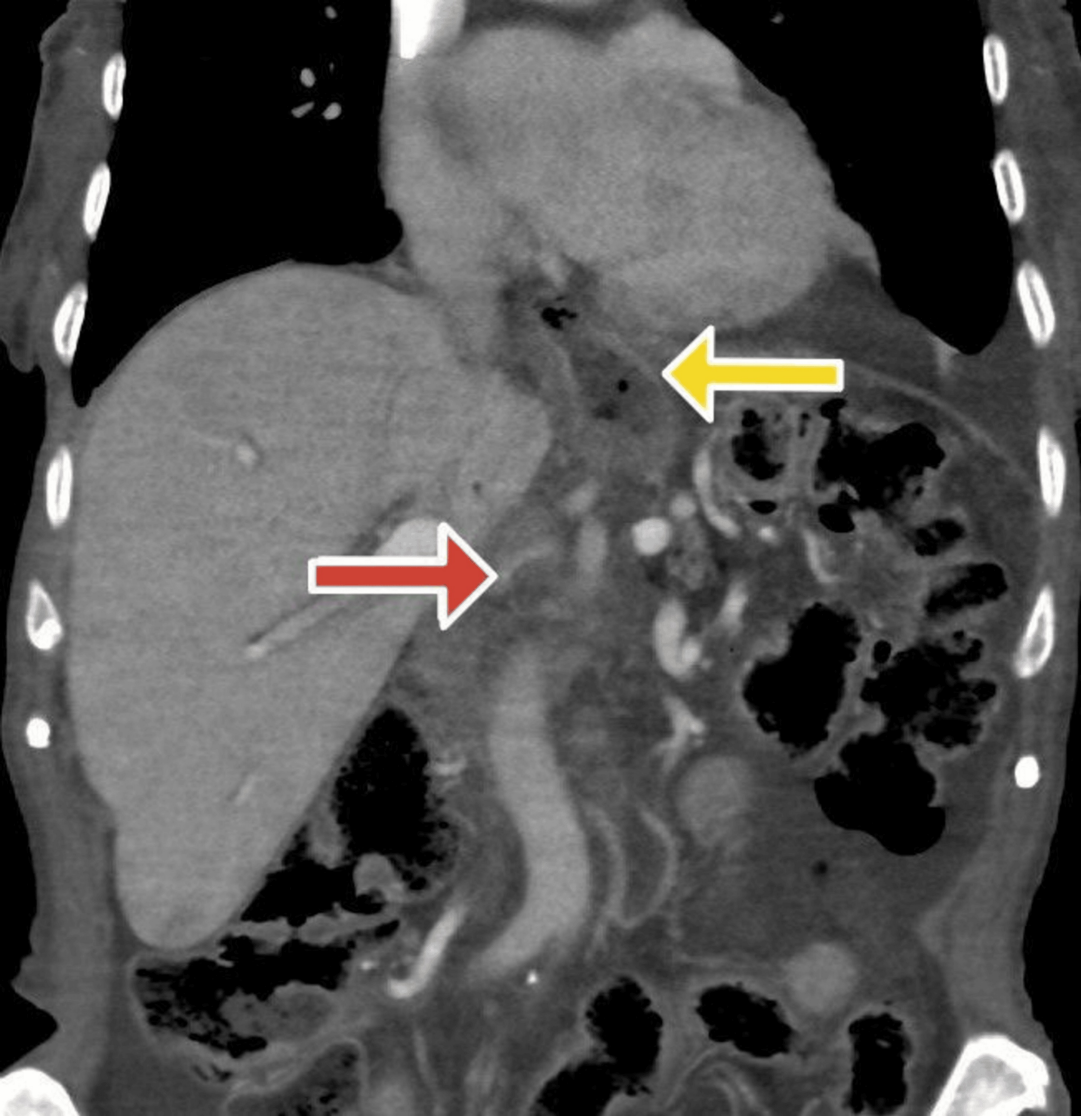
The CT scan displaying the preservation of the gastroduodenal artery (red arrow) with contrast enhancement of the stomach wall (yellow arrow), suggesting arterial supply from the RGEA.
